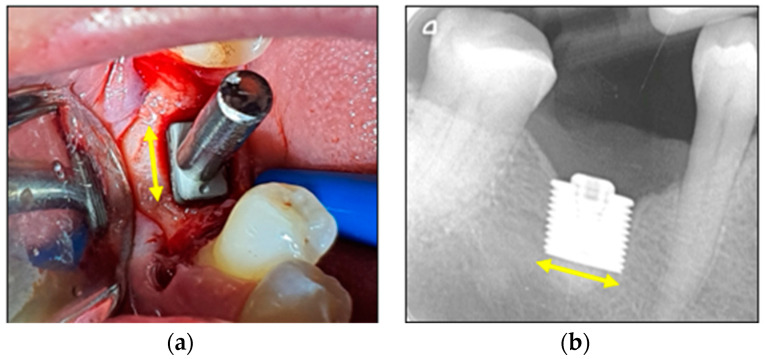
Patient 2. (a) Placement of the RBI into the osteotomy with a stem attached. Finger pressure on the stem confirmed excellent stability. (b) Radiographic image of the fully seated and well-stabilised RBI. Yellow arrows represent relative 6 mm lengths.
